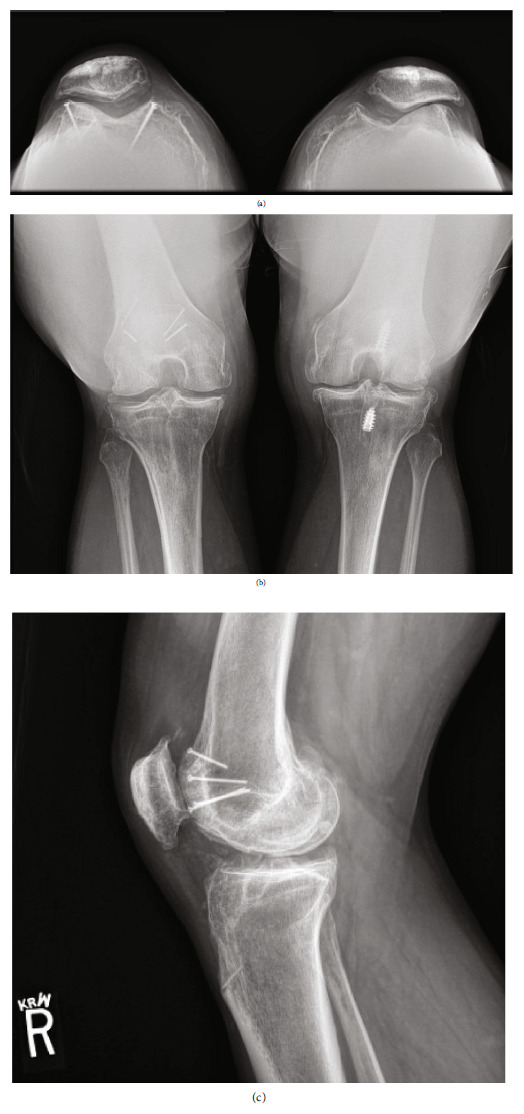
Eight-year postoperative radiographs. (a) Merchant's view demonstrating trochlear implant incorporation with maintained patellofemoral joint space on the right (KL-2) but progression of disease on the left (KL-4). (b)Posterior-anterior Rosenberg view demonstrating maintained medial/lateral joint spaces on the right (KL-2) with left knee progression (KL-4). (c) Right knee lateral radiograph demonstrating excellent trochlear allograft incorporation.
